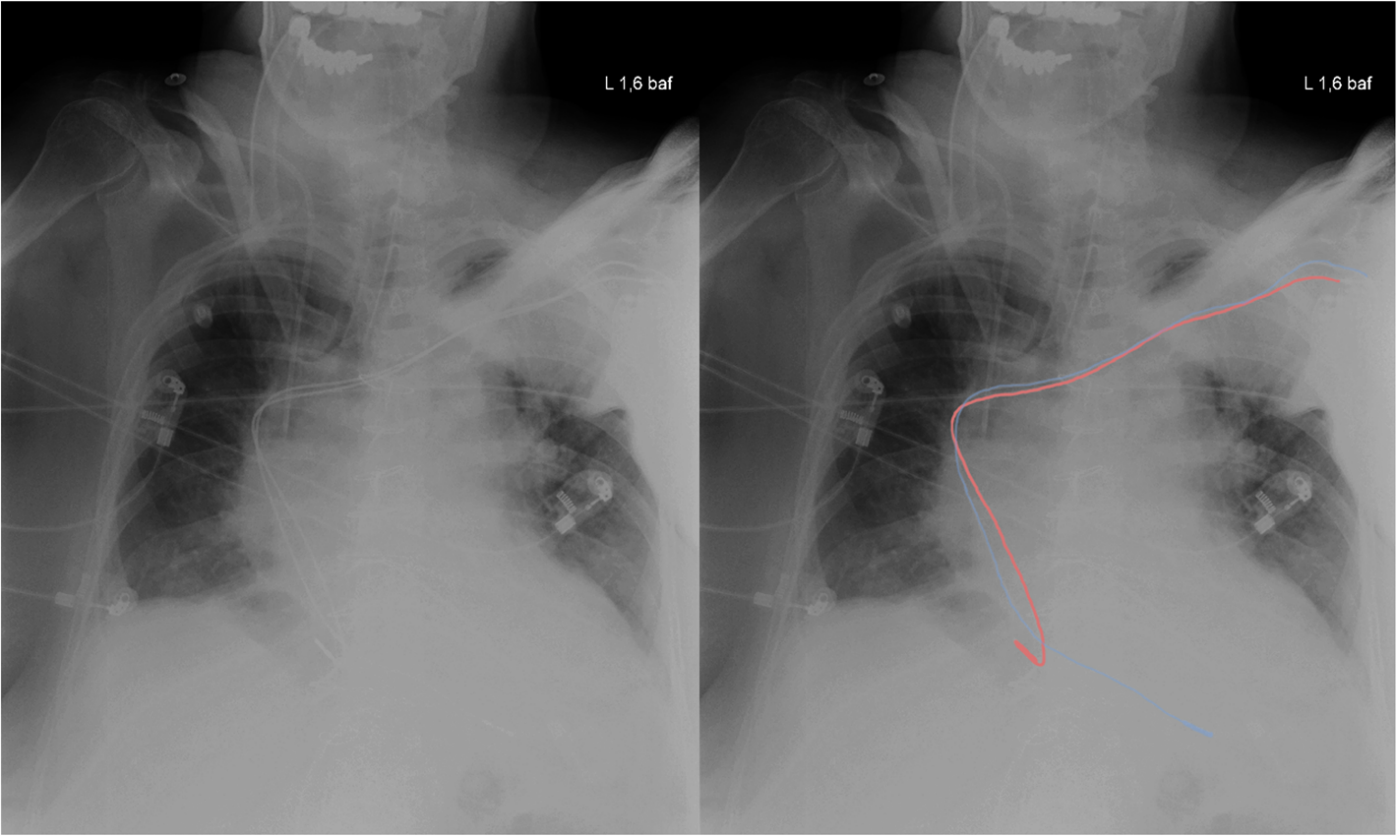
Post-operative chest X-ray. Both leads were re-positioned.
